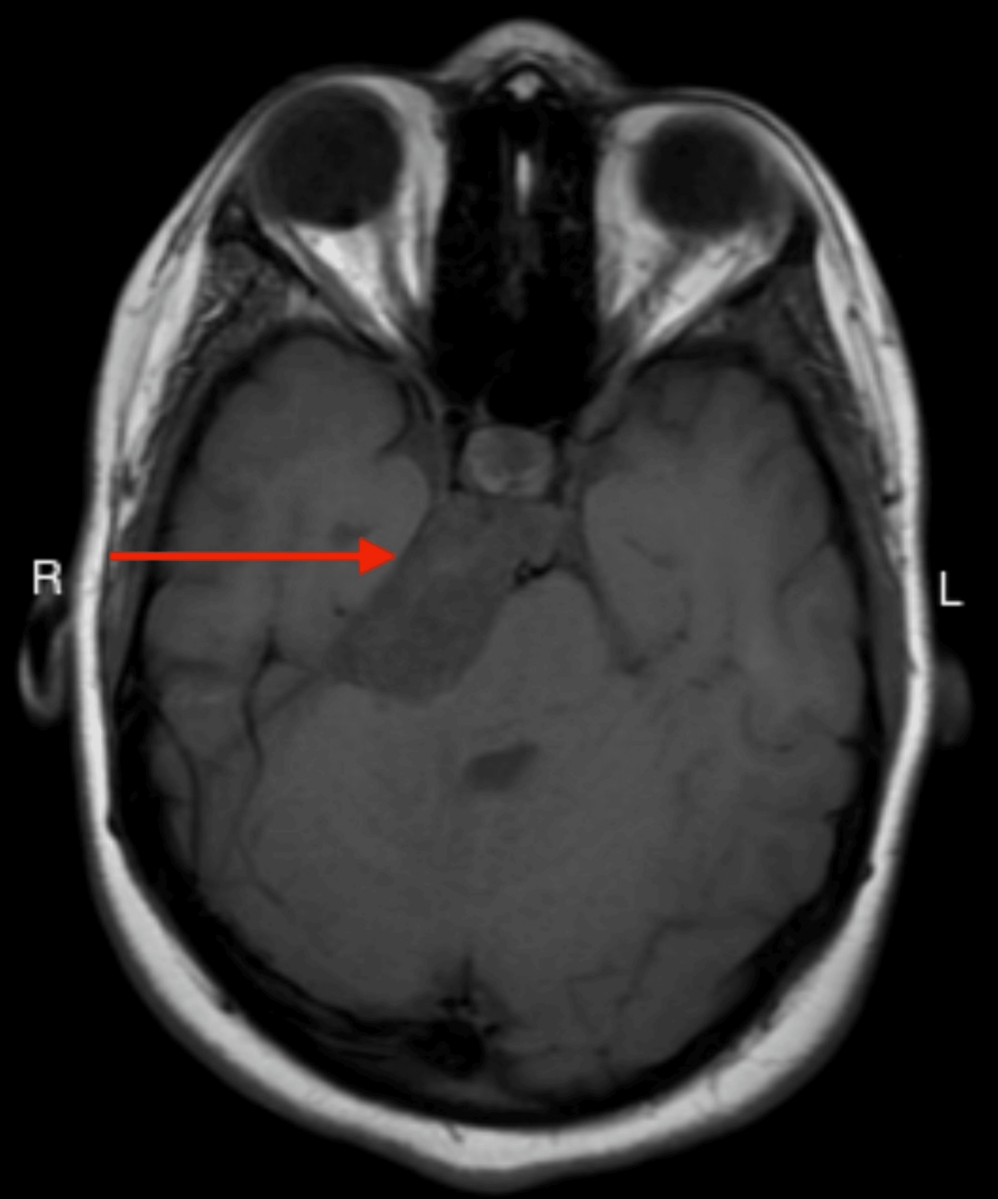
MRI of the brain Axial T1-weighted MRI image showing hypointense space-occupying lesion (arrow). MRI: magnetic resonance imaging.
